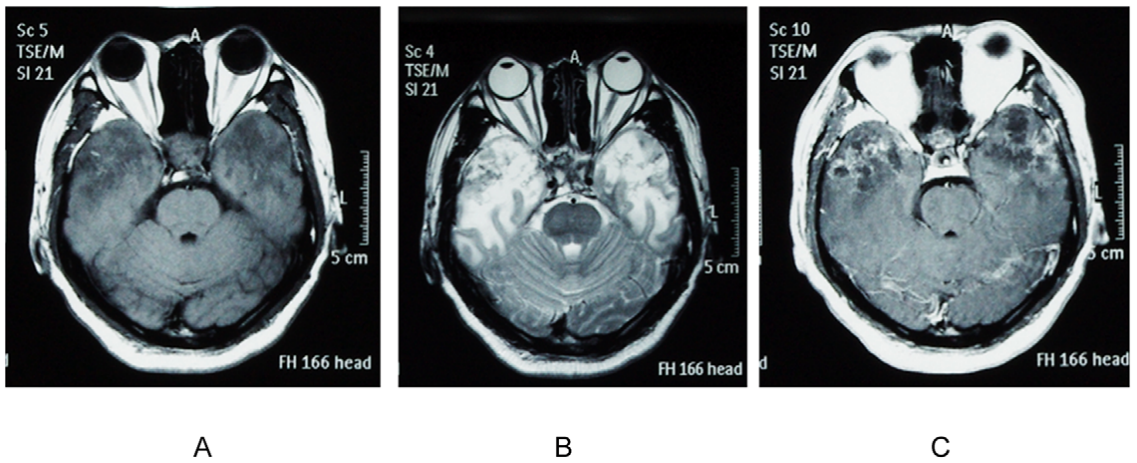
The brain MRI scan of a patient with RI. (A) The axial T1-weighted imaging showed relatively low-signal-intensity lesions in the bilateral temporal lobes. (B) The T2-weighted imaging revealed high-signal-intensity lesions in the bilateral temporal lobes. T1-weighted image after contrast administration (C) showed irregular edge contrast enhancement of the bilateral temporal lobes.
